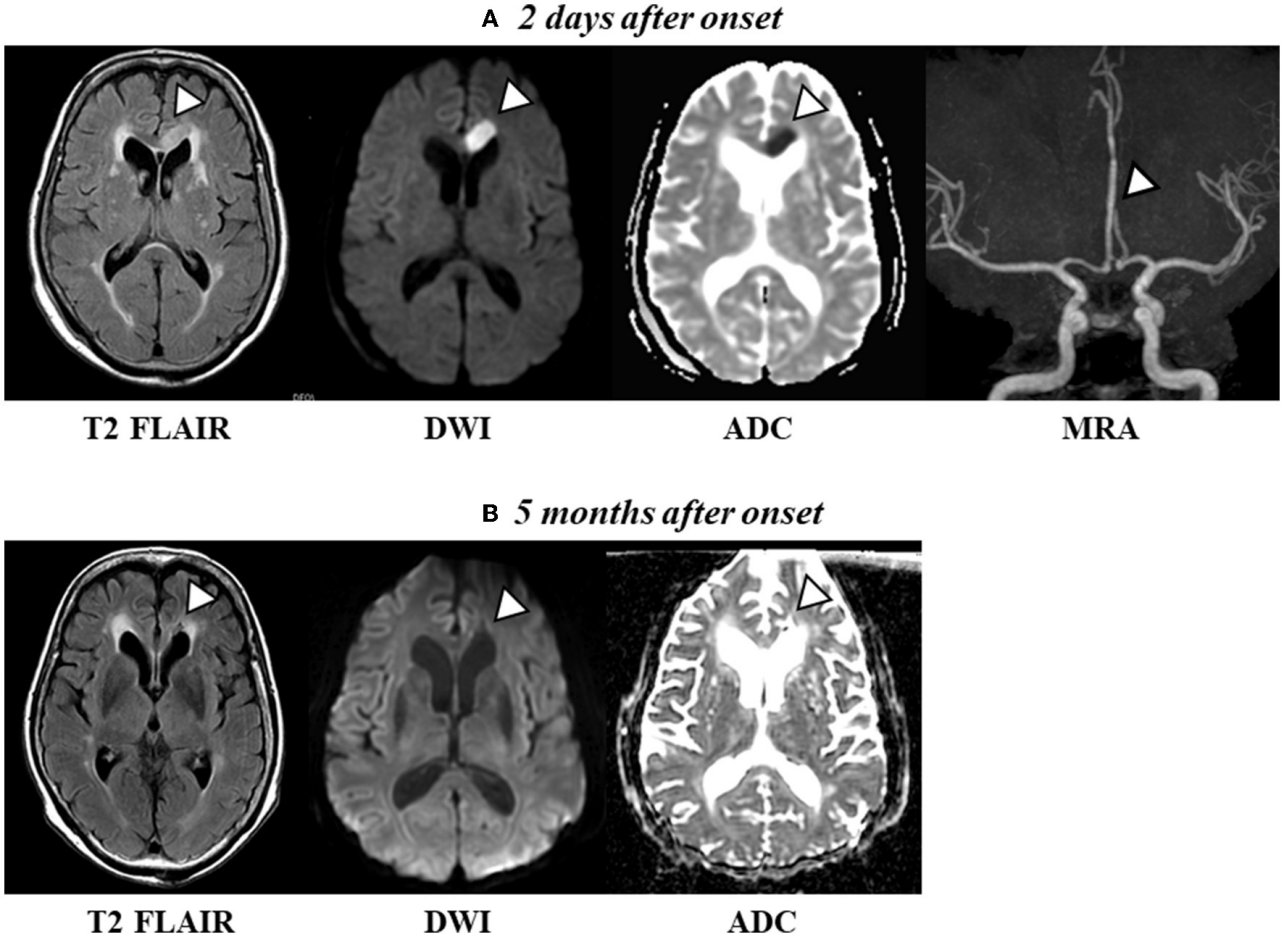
(A) Axial T2 FLAIR scan and diffusion-weighted imaging (DWI) revealed a high-intensity signal [apparent diffusion coefficient (ADC) shows a low signal intensity, indicating acute cerebral ischemia] in the left frontal lobe and midbody of the CC 2 days after onset. Magnetic resonance angiography (MRA) images revealed a vessel occlusion of the left A2. (B) Axial T2 FLAIR and DWI show a change to a low-intensity signal (conversely, ADC shows a high-intensity signal, indicating a chronic phase) in the left frontal lobe and the midbody of the CC 5 months after onset.
